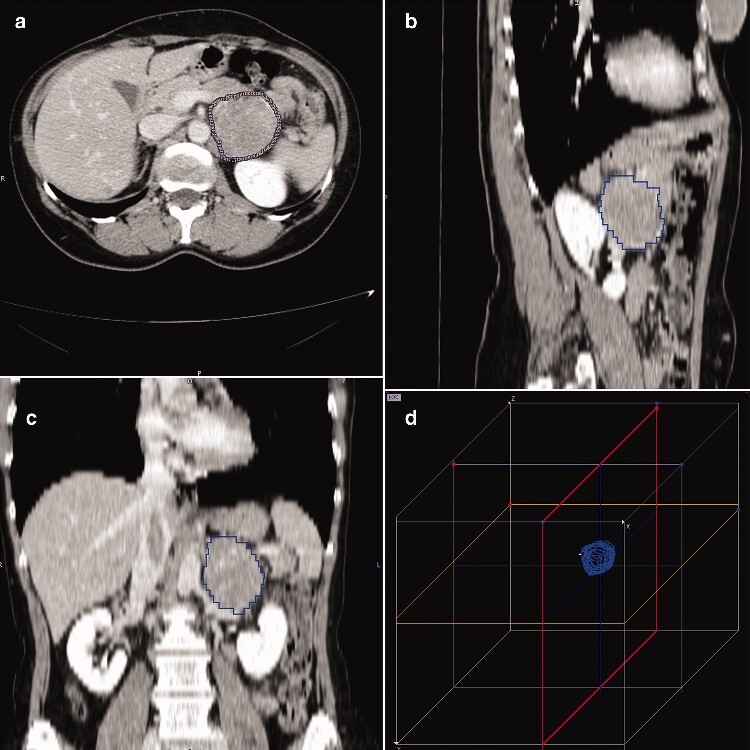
Volume of interest of an adrenal mass delineated using PMOD software Volume of interest of adrenal mass on venous-phase CT: a axial, b sagittal, and c coronal views, and d volume rendering reconstruction.
